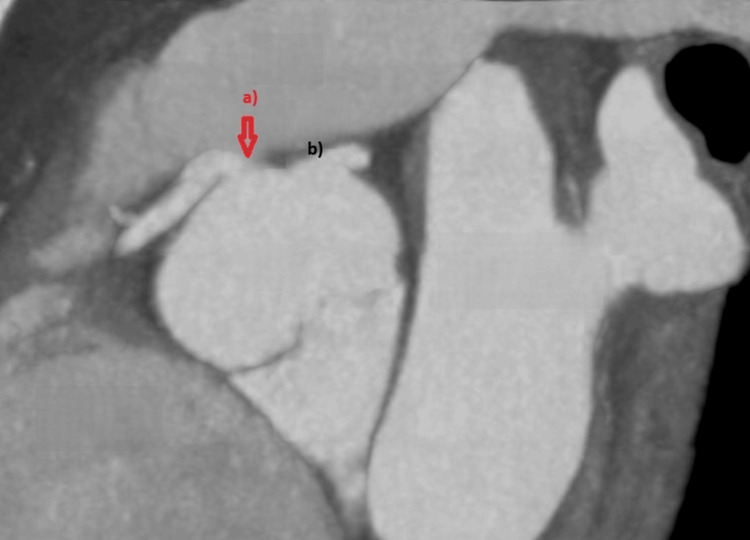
Multiplanar reconstruction of CCTA (oblique sagittal view) showing: (a) anomalous origin of the right coronary artery at the level of the aortic wall between the right and left coronary sinuses (red arrow); (b) left coronary artery origin from the left sinus. CCTA, coronary computed tomography angiography
